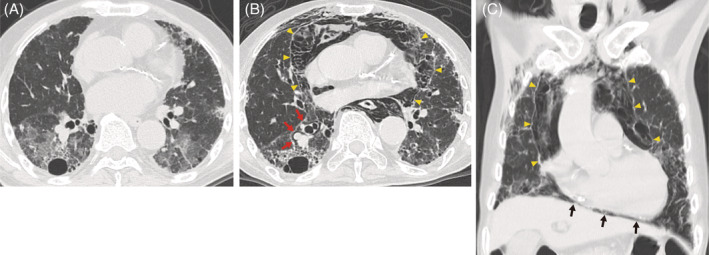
(A) Axial computed tomography (CT) at the onset of acute exacerbation of interstitial pneumonia showed bilateral ground‐glass opacities. (B) Axial CT on admission at the same level as (A) demonstrated massive pneumomediastinum (yellow arrow heads) with compression of the cardiovascular structures and air along the peribronchovascular bundle (red arrows). (C) Coronal section of the pneumomediastinum (yellow arrow heads) revealed a continuous diaphragm sign (black arrows)
